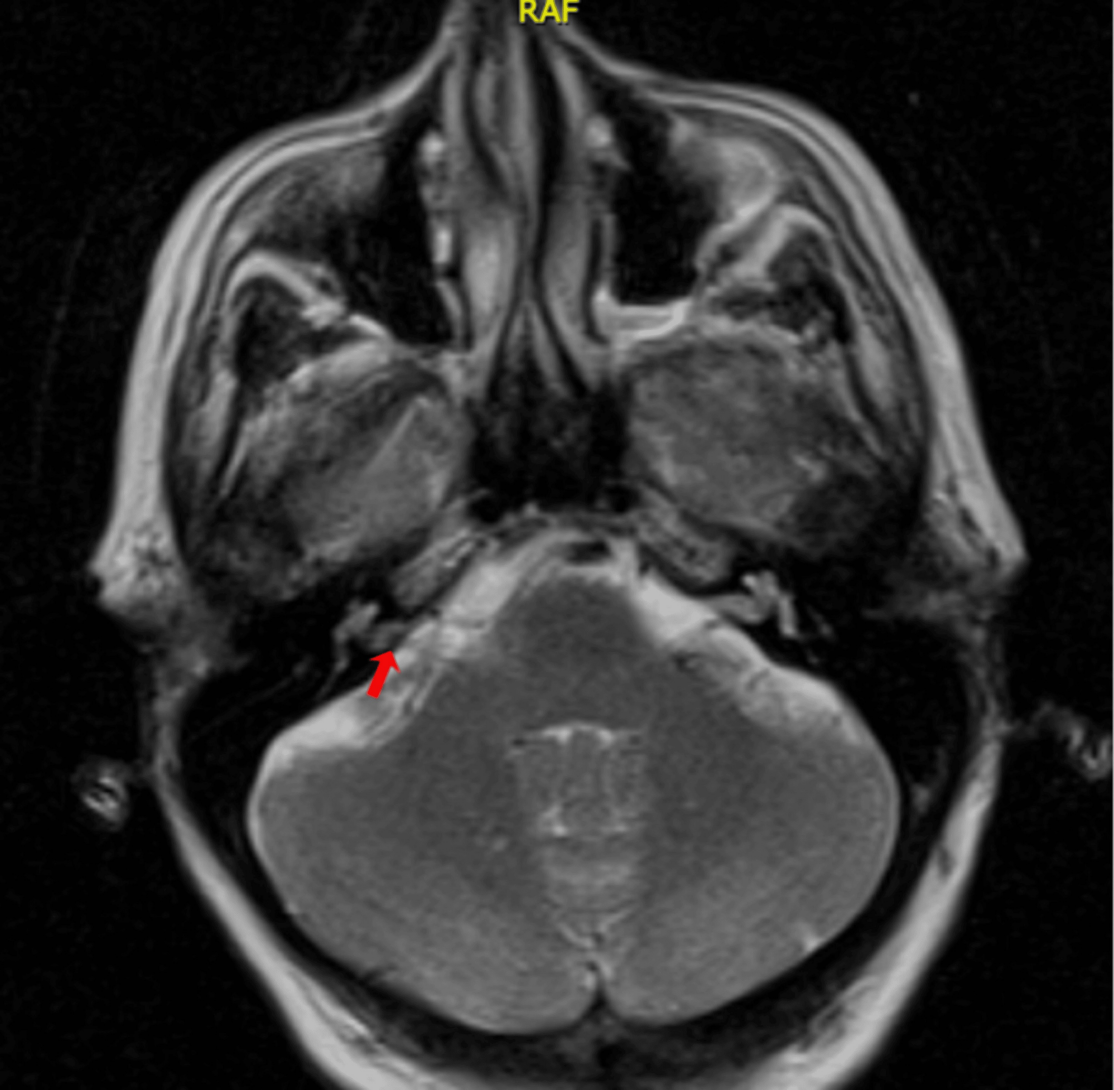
Axial T2-weighted brain MRI of patient no.1. Red arrow: Right-sided neurovascular compression (Chavda classification grade I)
